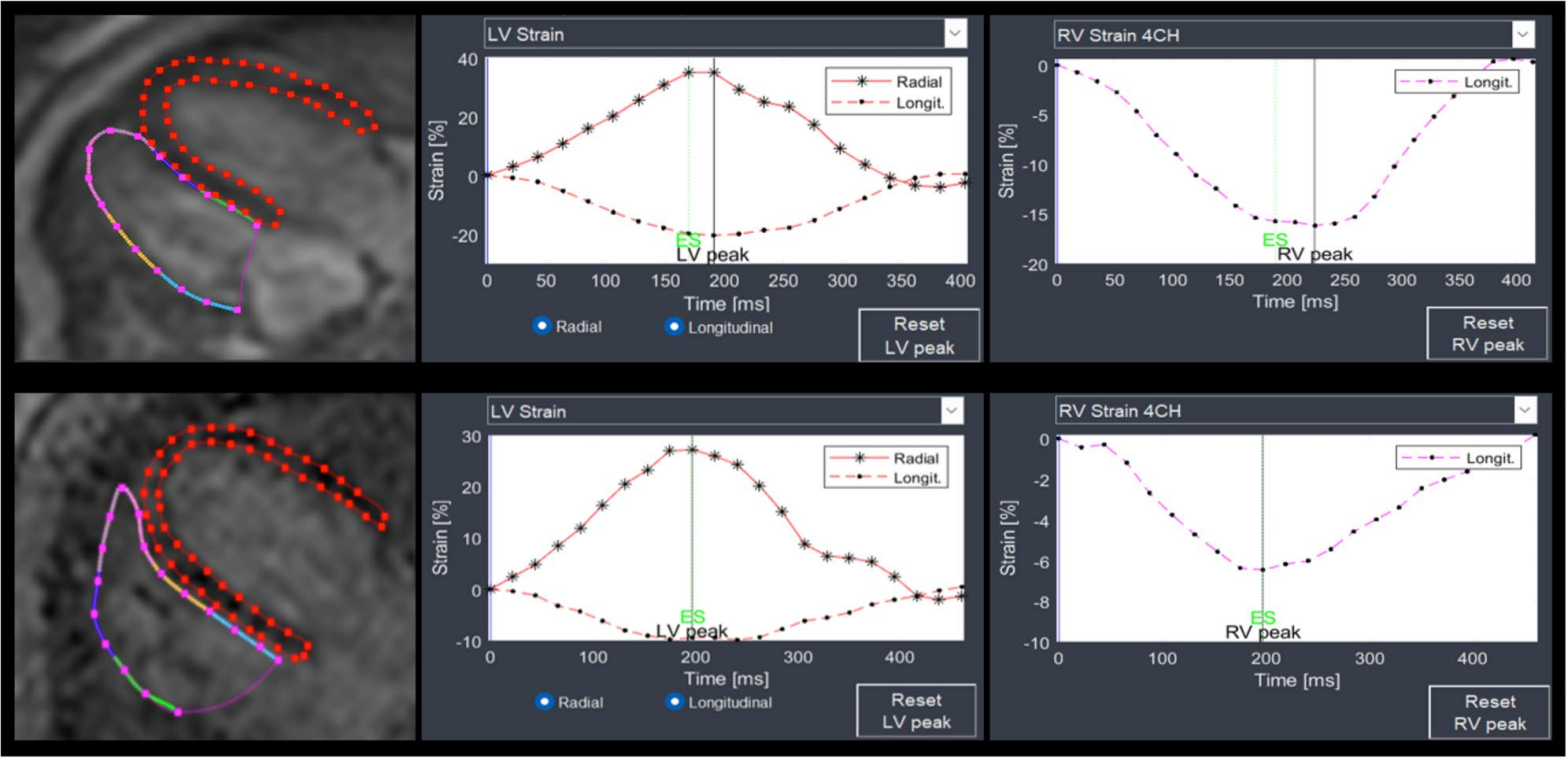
Assessment of strain parameters from fetal cardiac MRI cine 4-chamber views. Upper row: Contouring of endo- and epicardial boundaries of the left ventricle (LV, red) and the right ventricle (RV, colored line) with inclusion of the tricuspid valve plane in fetal cardiac MRI end-diastolic 4-chamber view of a healthy fetus (gestational age, 36^+6^ weeks). The software automatically propagates defined contours to calculate LV and RV strain parameters: LV GLS − 20.5%, RV GLS − 17.1%, radial strain 34.9%, strain rate 1.70 s^−1^. Bottom row: Fetal cardiac MRI end-diastolic 4-chamber view with LV and RV contouring in a fetus with CHD (diagnosis: TGA, gestational age, 36^+2^ weeks). LV and RV GLS and strain rate were increased (i.e., less negative) while radial strain was normal: LV GLS − 9.4%, RV GLS − 6.5%, radial strain 27.2%, strain rate 0.84 s^−1^
